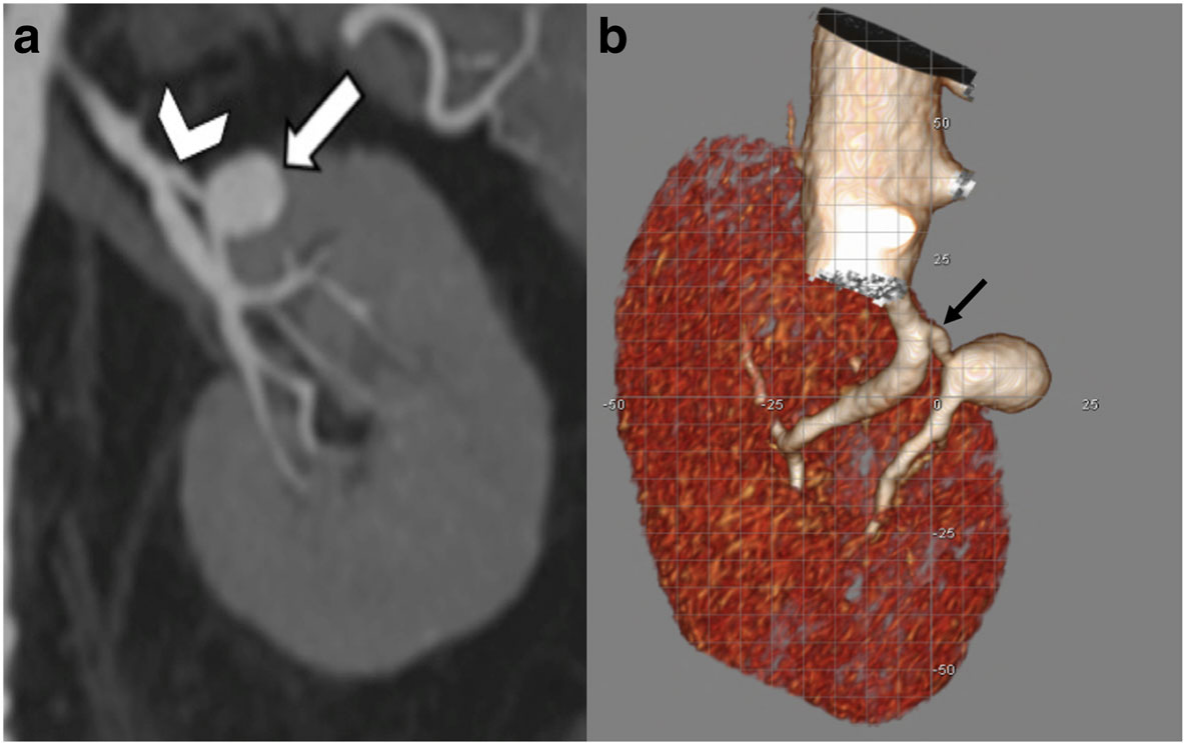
a CTA demonstrating a solitary left kidney with a wide-necked 2 cm saccular aneurysm (arrow) arising from the left renal artery anterior division (arrow head). b Volume-rendered 3D CTA reconstruction demonstrating the left TRAA morphology arising from the anterior division (arrow) just distal to the left renal artery bifurcation
